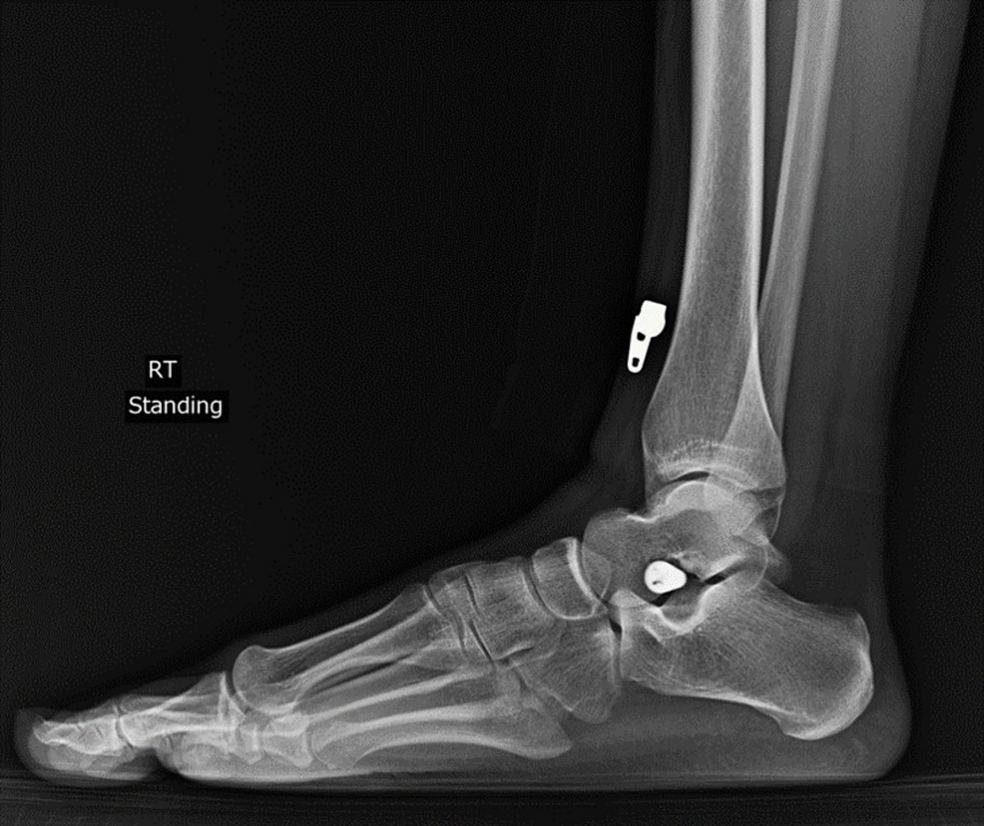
Postoperative lateral right foot X-ray with sinus tarsi implant.
